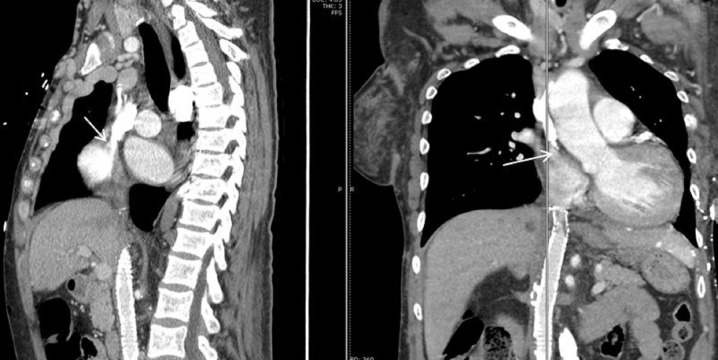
CT chest with contrast showing significant narrowing of the superior vena cava at the right atrial junction (white arrow)
